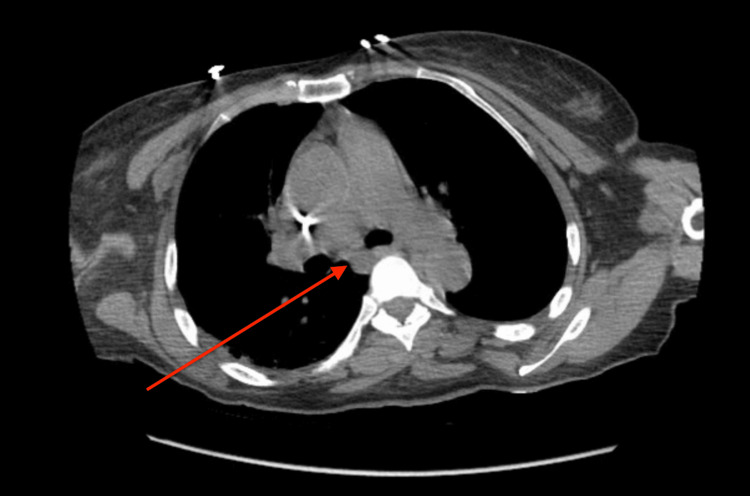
CT scan of the chest without contrast The image is showing no hilar lymphadenopathy (as demonstrated by the red arrow).
